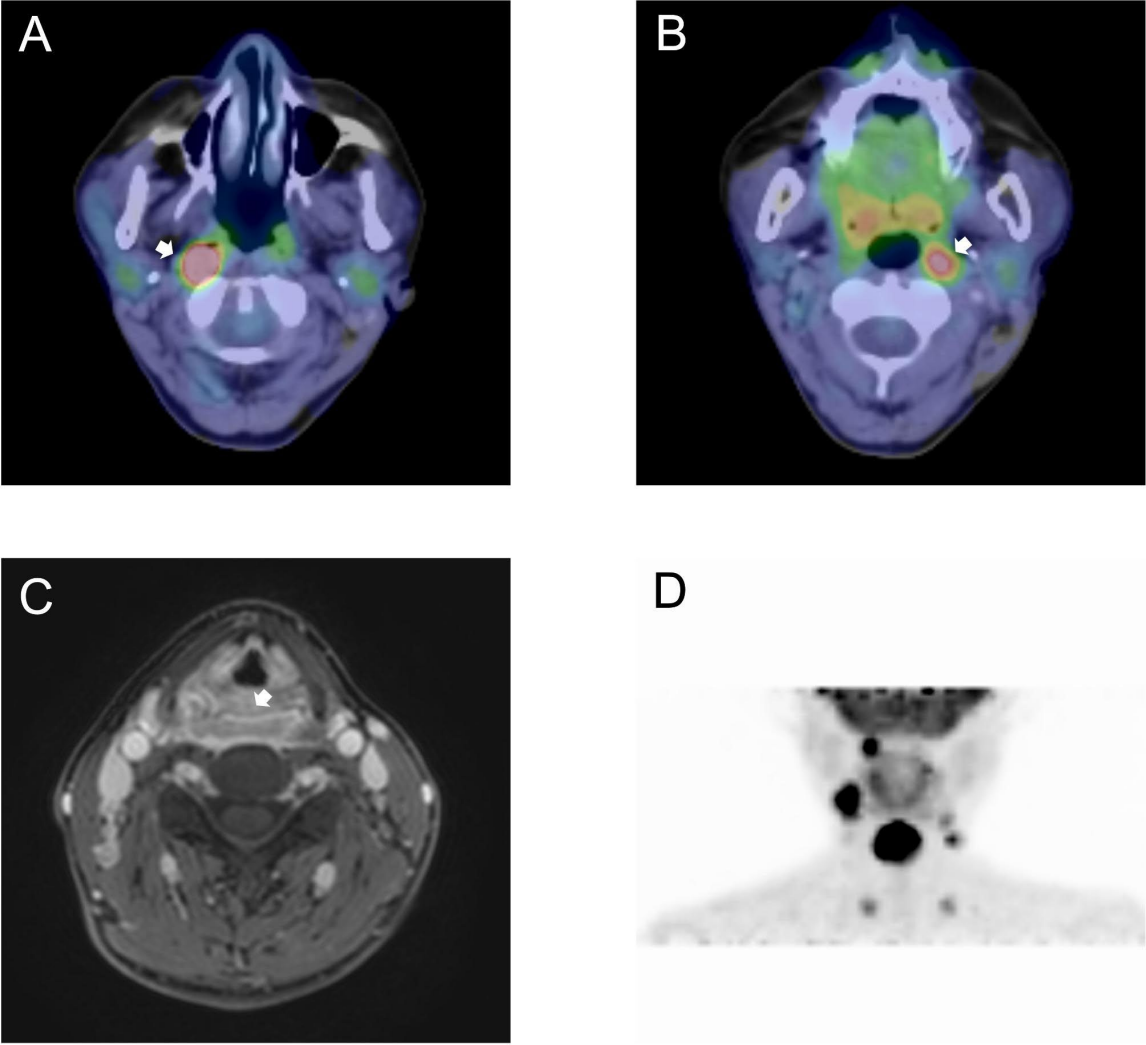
Hypopharyngeal carcinoma with bilateral retropharyngeal lymph node metastasis (RPLNM). (A) [^18^ F]-fluoro-2-deoxy-D-glucose (FDG)-positron emission tomography (PET)/computed tomography (CT)-fused images show right RPLNM (arrow; longest diameter = 14 mm, SUV_max_ = 11.4). (B) FDG–PET/CT-fused images show left RPLNM (arrow; longest diameter = 9 mm, SUV_max_ = 6.7). (C) Contrast-enhanced fat-saturated eTHRIVE images of the posterior wall primary tumor predominantly on the right side. (D) FDG–PET image revealed bilateral cervical LNM
